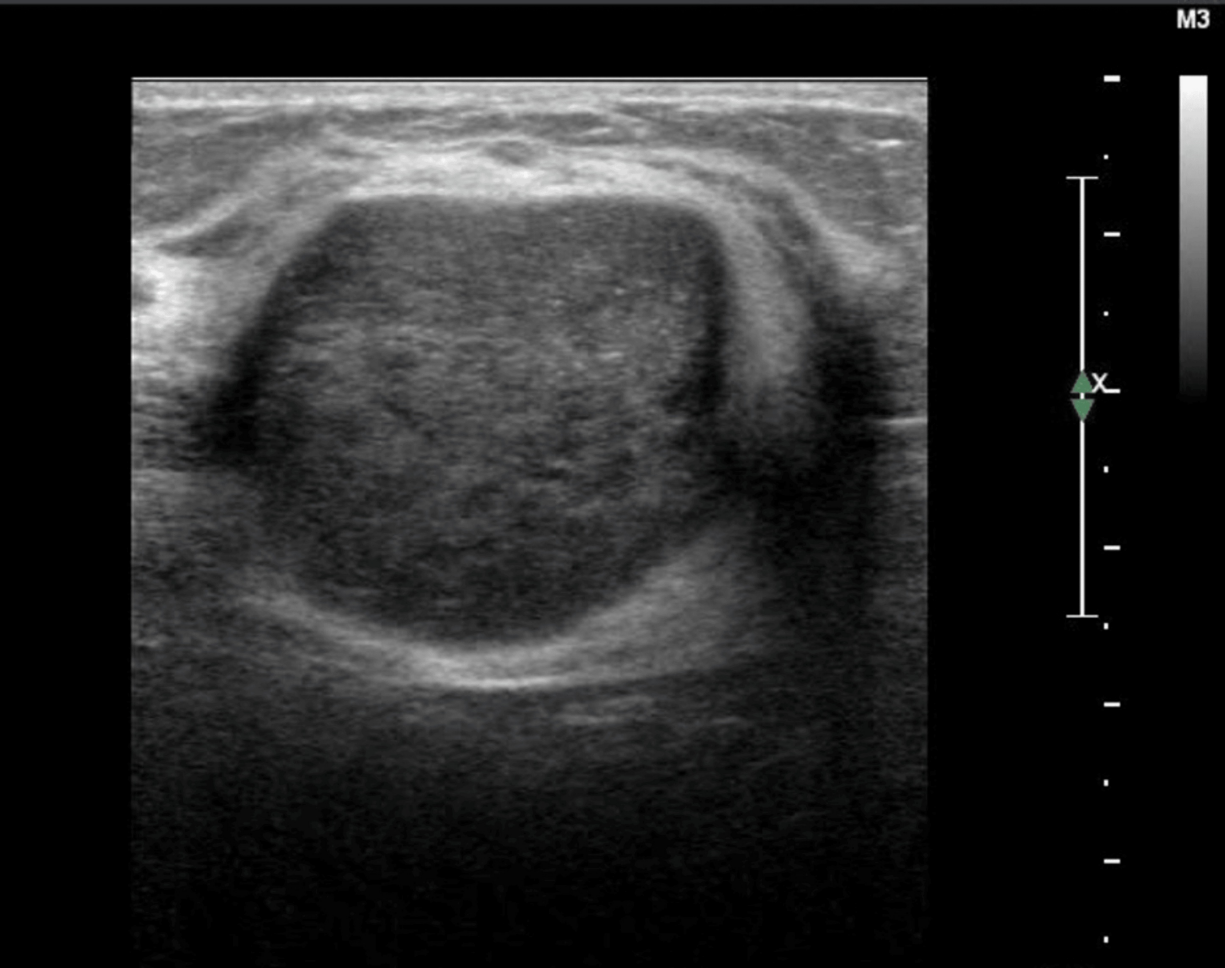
HHUS image of a 30-year-old patient The hand-held ultrasound. (HHUS) image is showing well-circumscribed hypoechoic lesion with a smooth margin at the 12 o'clock position of the left breast.
